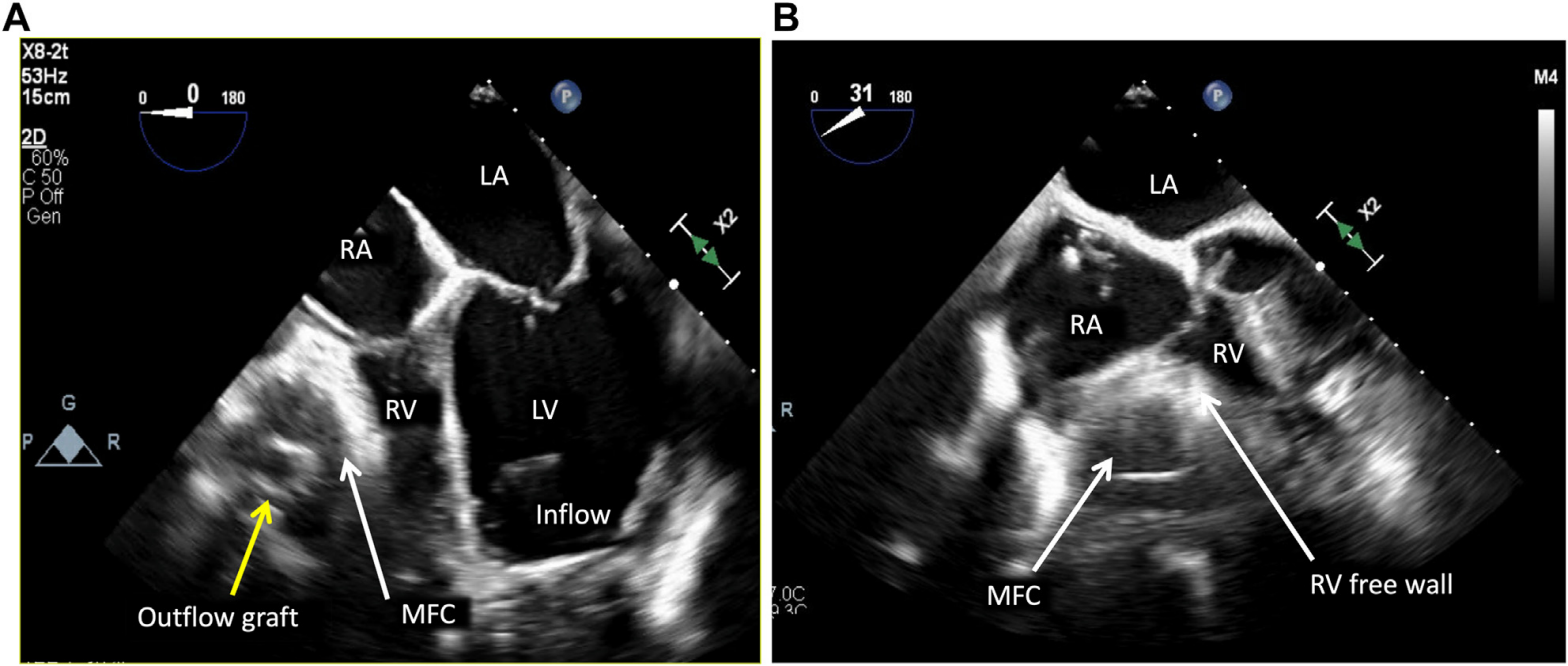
Two-dimensional transesophageal echocardiography, midesophageal long-axis (A; 0°) and oblique long-axis (B; 31°) views, demonstrates compression of the right ventricular free wall by an MFC *(white arrow)* surrounding the outflow graft *(yellow arrow)* and abutting the right ventricular free wall. *LA*, Left atrium; *LV*, left ventricle; *RA*, right atrium.
